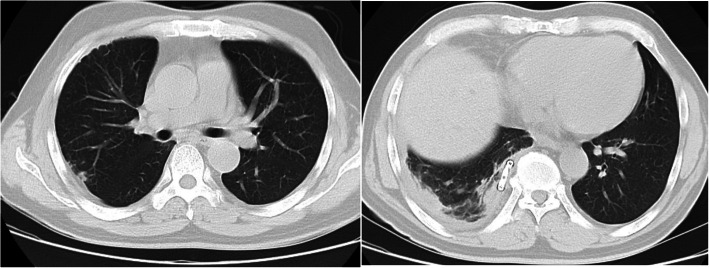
Chest computed tomography scan revealing right-sided pleural effusion and pulmonary infection. Date: October 11, 2018
